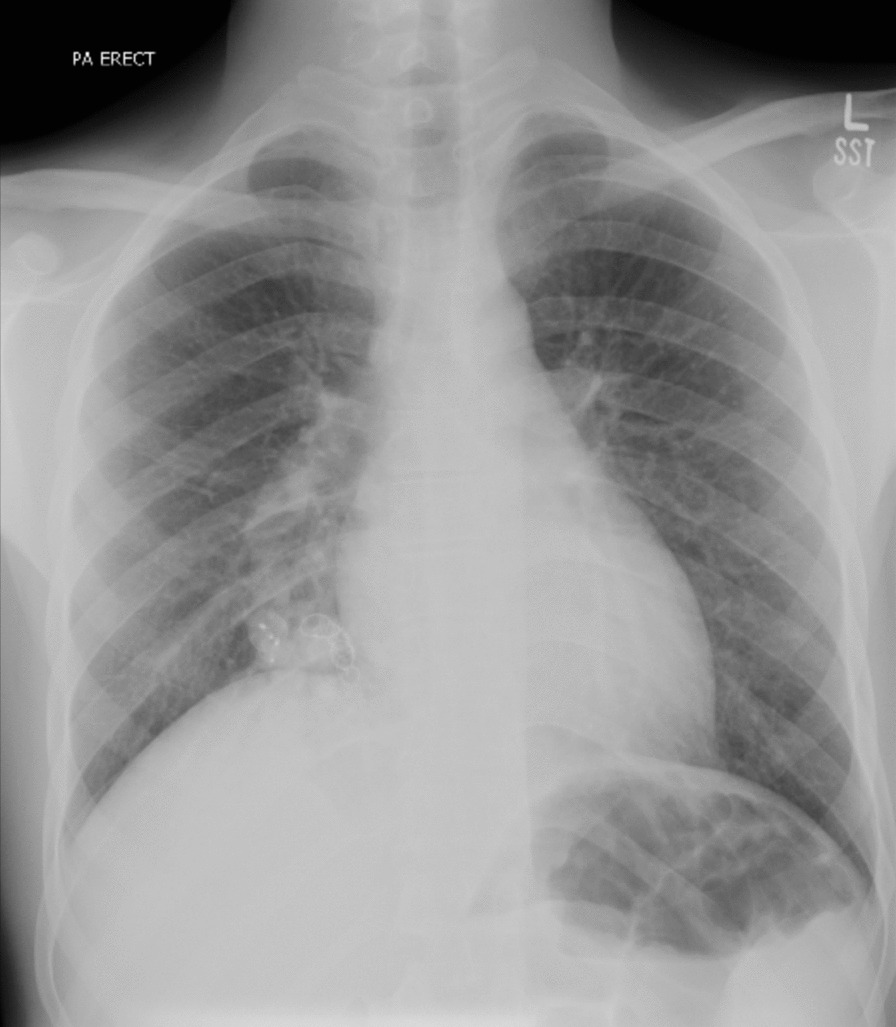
Chest X-ray at 1-week follow-up. Note the increased opacity around the cardiac silhouette at the site of angioembolization
